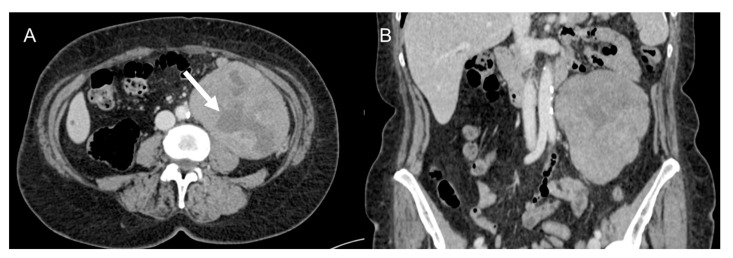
Retroperitoneal leiomyosarcoma in a 63-year-old woman. Axial (A) and coronal (B) CT images on venous phase show a 10.2 cm heterogeneous mass with central area of low attenuation consistent with necrosis (arrow).
